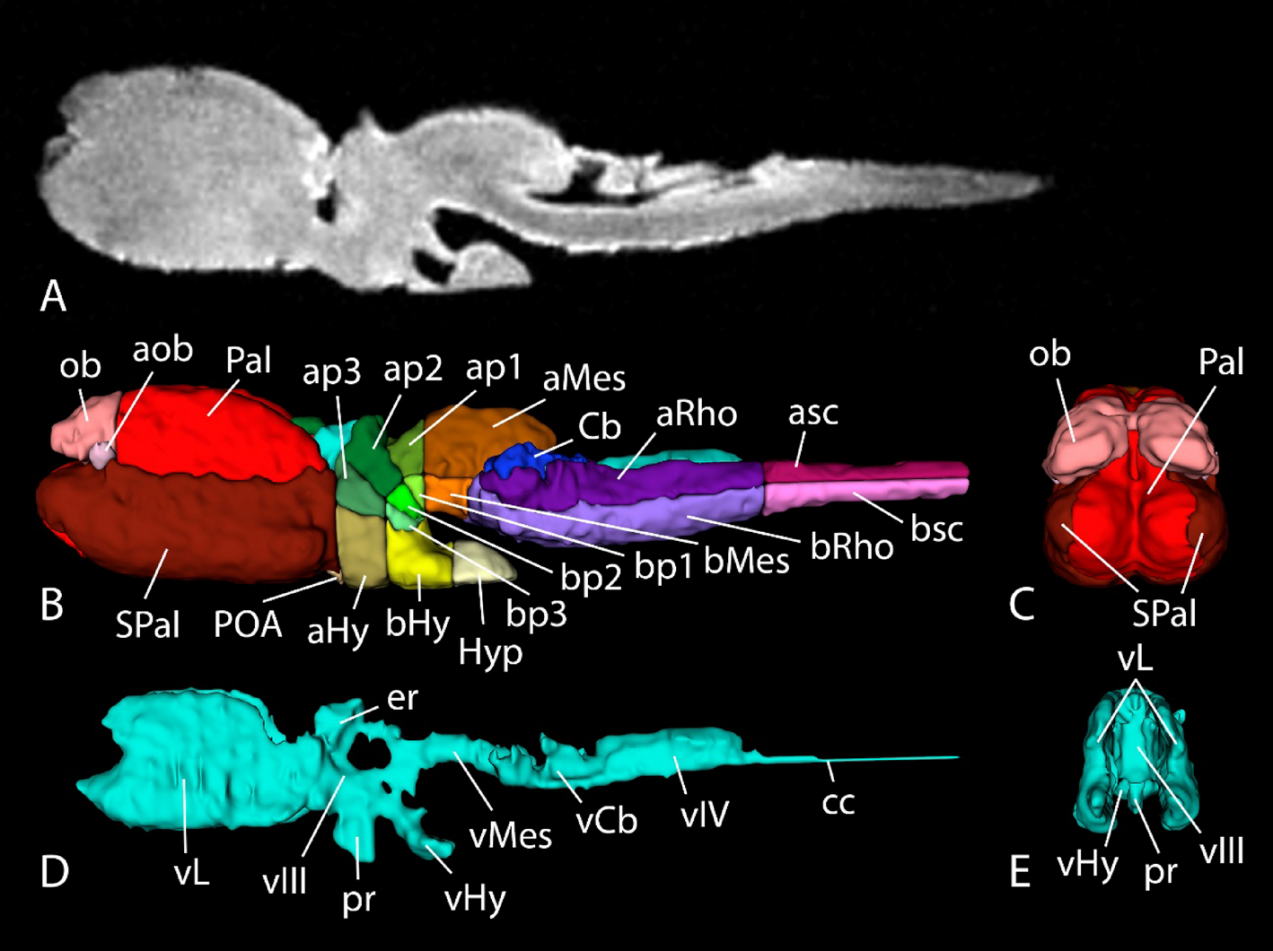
MR image (A) and 3D reconstructions of the brain (B,C) and the ventricular system (D,E) of *Protopterus annectens* in sagittal (A,B,D) and frontal (C,E) views. *aHy* alar hypothalamus, *aMes* alar mesencephalon, *aob* accessory olfactory bulb, *ap1* alar part of p1, *ap2* alar part of p2, *ap3* alar part of p3, *aRho* alar rhombencephalon, *asc* alar spinal cord, *bHy* basal hypothalamus, *bMes* basal mesencephalon, *bp1* basal part of p1, *bp2* basal part of p2, *bp3* basal part of p3, *bRho* basal rhombencephalon, *bsc* basal spinal cord, *Cb* cerebellum, *cc* central canal, *er* epithalamic recess, *Hyp* hypophysis, *ob* olfactory bulb, *Pal* pallium, *POA* preoptic area, *pr* preoptic recess, *SPal* subpallium, *vCb* cerebellar ventricle, *vHy* hypothalamic ventricle, *vIII* third ventricle, *vIV* fourth ventricle, *vL* lateral ventricles, *vMes* mesencephalic ventricle.
